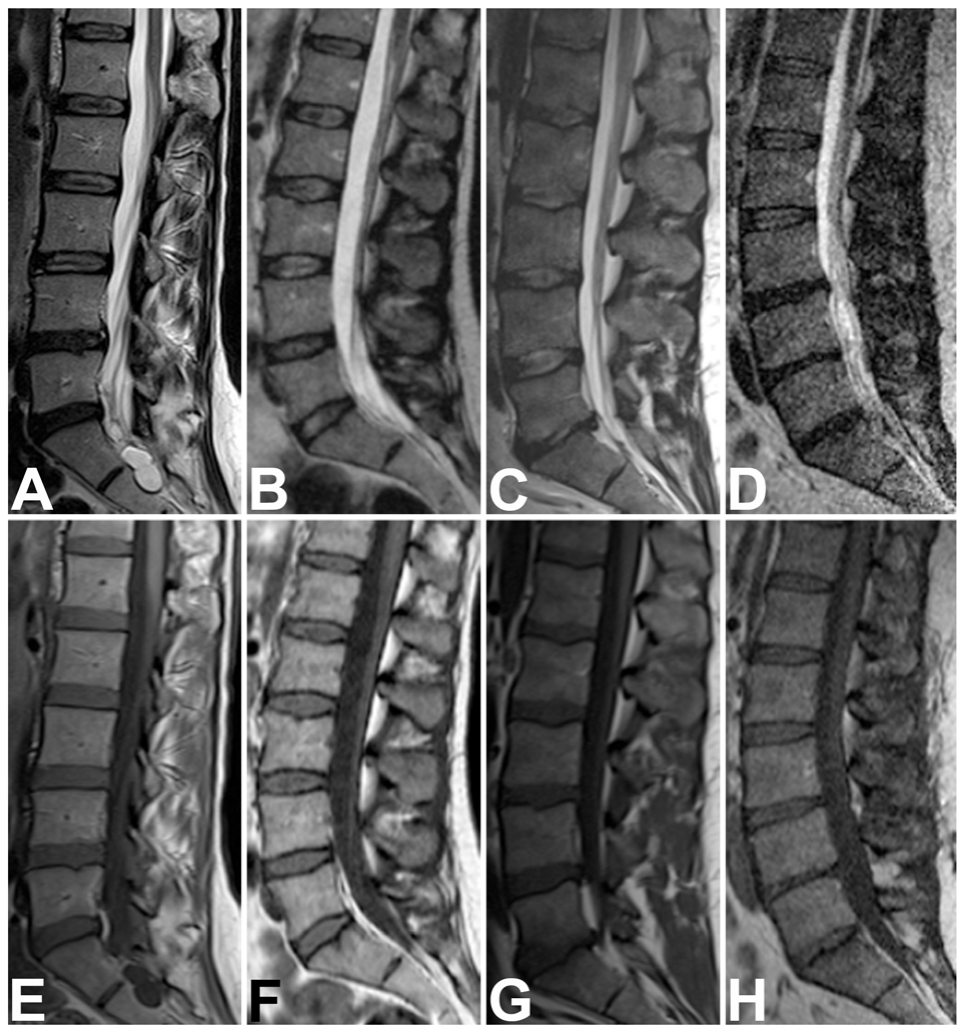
Representative Examples of Image Quality on Sagittal T2- and T1-Weighted Sequences of the Lumbar Spine. Top row: sagittal T2-weighted turbo spin-echo sequences of the lumbar spine of four patients, representing the range of qualitative image quality scores obtained with short-bore (A, C) and open MR imaging (B, D). Overall image quality ratings were: “optimal” for A,“good” for B, “moderate” for C, and “poor” for D. No sequence was rated very poor (nondiagnostic). Bottom row: sagittal T1-weighted turbo spin-echo sequences of the lumbar spine of the same four patients obtained with short-bore (E, G) and open MR imaging (F, H). Overall image quality ratings were: “good” for sequence E,“moderate” for F, “good” for G, and “poor” for H. No sequence was rated very poor (nondiagnostic).
